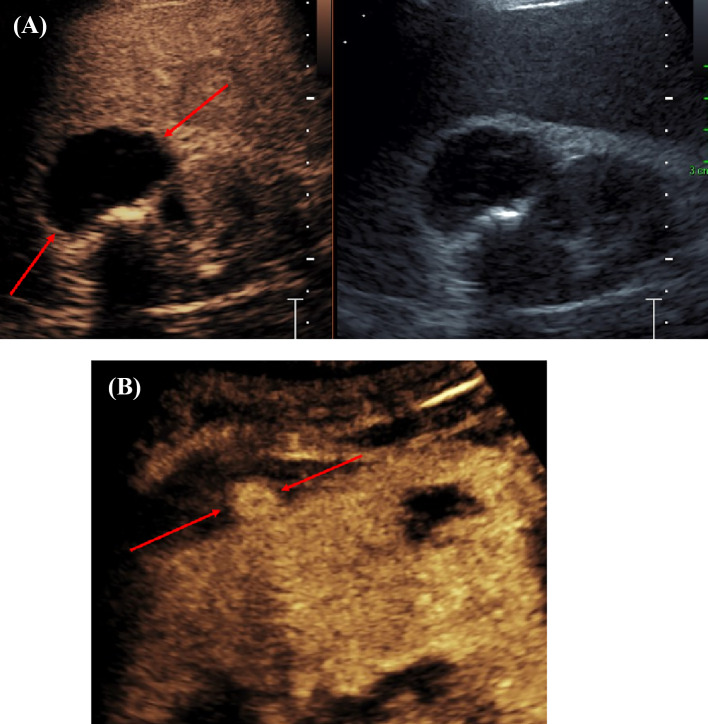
60-year-old male with a recurrence 6 years after partial nephrectomy for an oncocytic RCC. The CEUS is a dual screen image with the contrast enhanced ultrasound on the left and a synthesized B-mode image on the right. The B-mode image is used to confirm localization and is not a diagnostic image. The initial CEUS post partial nephrectomy (A) demonstrates no enhancement at the surgical site (red arrows). On follow-up CEUS 6 years (B) later there is a 1.0 cm enhancing nodule (red arrows). CECT or CEMRI were not performed. The patient remains in monitoring
